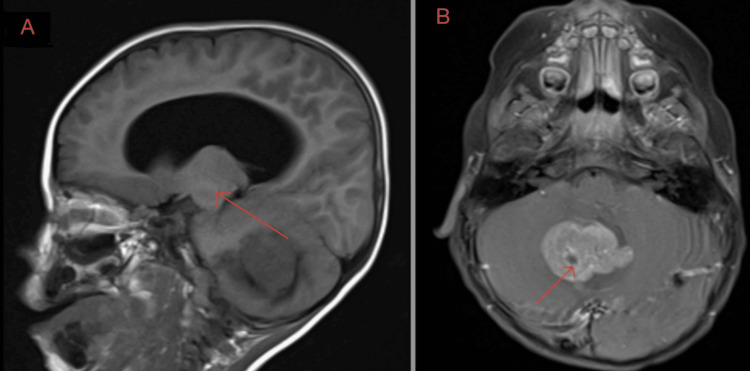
MRI demonstrating posterior fossa mass with obstructive hydrocephalus (A) Sagittal MRI showing a posterior fossa mass arising from the cerebellar vermis (red arrows) with compression of the fourth ventricle. (B) Axial MRI demonstrating the same lesion (red arrows) with associated ventricular enlargement consistent with obstructive hydrocephalus. These findings were identified during the patient’s initial diagnostic workup. MRI: Magnetic resonance imaging.
